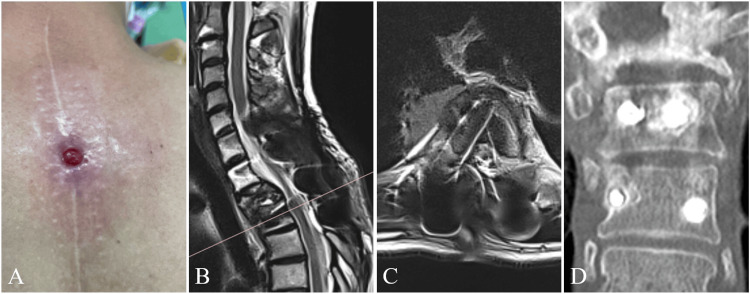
Cutaneous findings and radiological findings at the time of presentation MRI demonstrated a sinus tract (fistula) with high signal intensity on T2-weighted images, extending from the cutaneous lesion to the right T3 pedicle screw. CT images revealed a slight radiolucent halo around the screw in the right T4 and left T3 pedicles. (A) Cutaneous appearance, (B) T2-weighted sagittal image, (C) T2-weighted axial image, (D) CT coronal image. MRI: magnetic resonance imaging, CT: computed tomography
